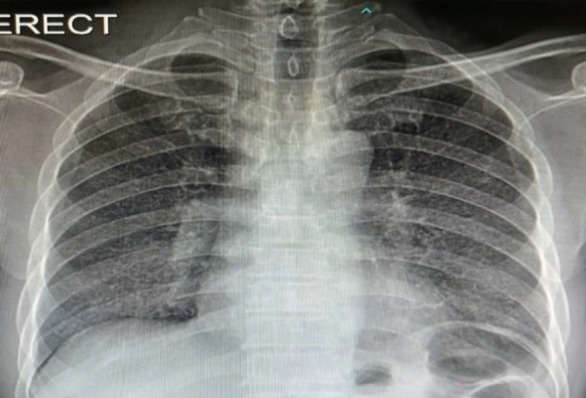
Normal chest x-ray without feature of tuberculosis
